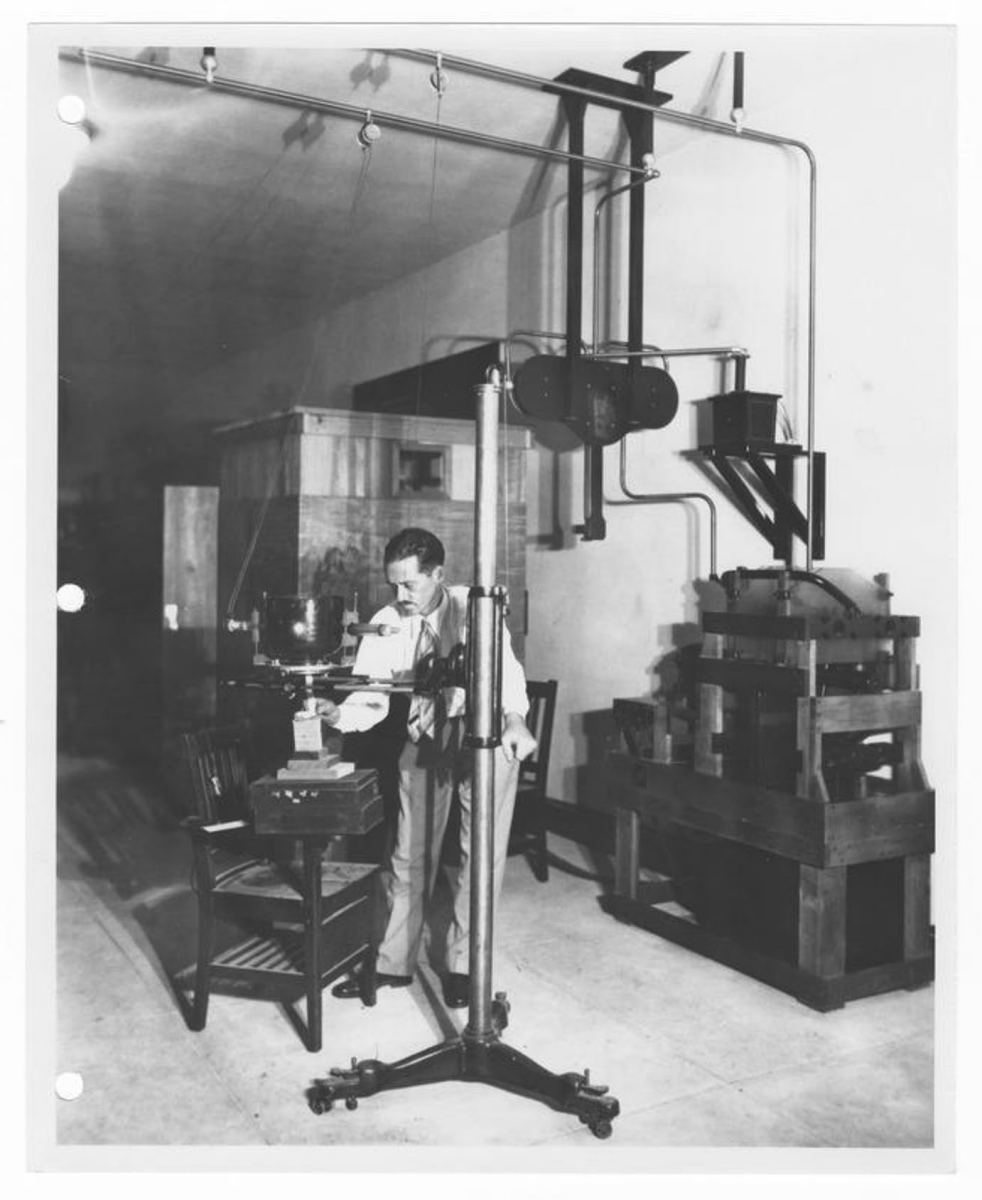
Muller’s graduate student, Clarence Paul “Pete” Oliver, working the X-ray machine at the University of Texas in 1927. Courtesy Helen Muller and Lilly Library, Indiana University, Bloomington, Indiana
